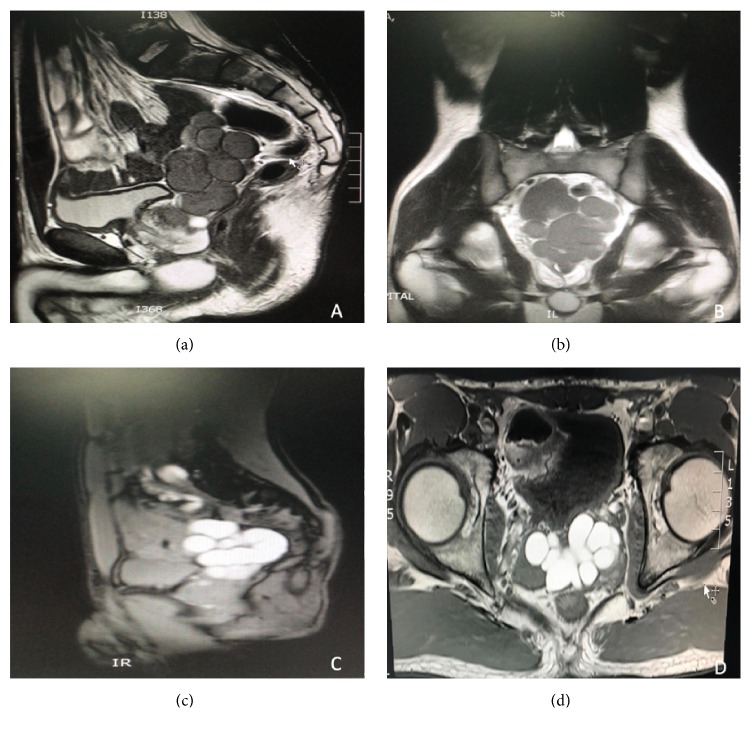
(a) MRI sagittal section T2, (b) MRI coronal section T2, (c) MRI sagittal section T1, and (d) MRI axial section T1. (a)-(b) showed diffuse dilatation of left seminal vesicles with low signal intensity, and (c)-(d) showed diffuse dilatation of left seminal vesicles with high signal intensity.
